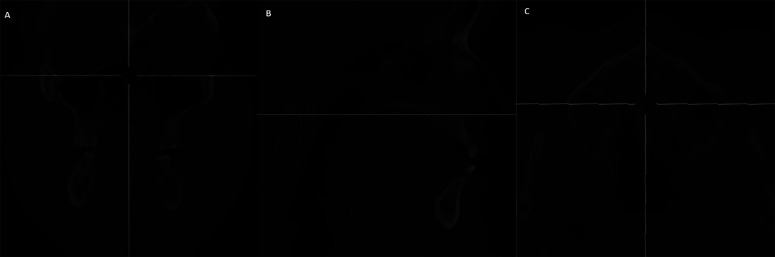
Standardization of CBCT image positioning: (A) Coronal view, (B) Sagittal view, and (C) Axial view.
